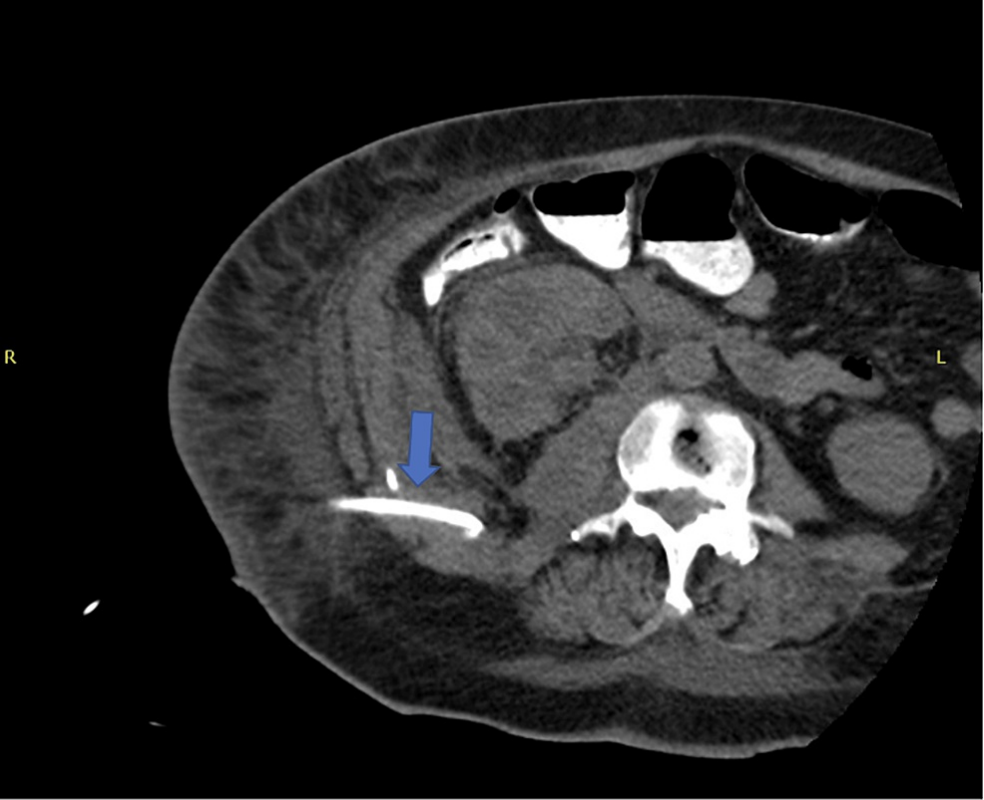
Imaging during computed tomography-guided drainage shows catheter placement in the center of the abscess, with a significant decrease in abscess volume (arrow)
